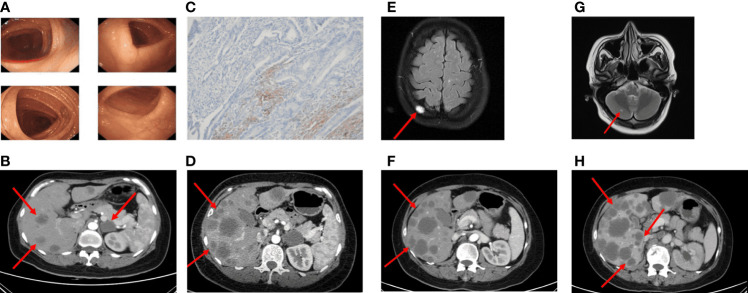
Imaging in the palliative care process CT revealed metastatic lesions. (A) Colonoscopy found no abnormal changes (2020-08). (B) Imaging examinations performed more than 2 years after surgery (2021-02). Multiple metastatic lesions in the liver. (C) The PD-L1 IHC suggested the PD-L1 expression was 2%. (D) After four cycles of FOLFIRI plus cetuximab treatment, CT scans revealed significant enlargement of liver metastasis (2021-04). (E) MRI scans after five cycles of FOLFIRI plus bevacizumab showed the new abnormal high density shadow of the right skull, considering metastasis (2021-06). (F, G) After two months of the therapy of mFOLFOX6 and palliative radiation, hepatic metastatic lesions on CT were similar to the previous, the cranial MRI suggested cerebellar metastasis (2021-09). (H) The patient's last CT reexamination showed liver metastasis progressed again (2021-12).
